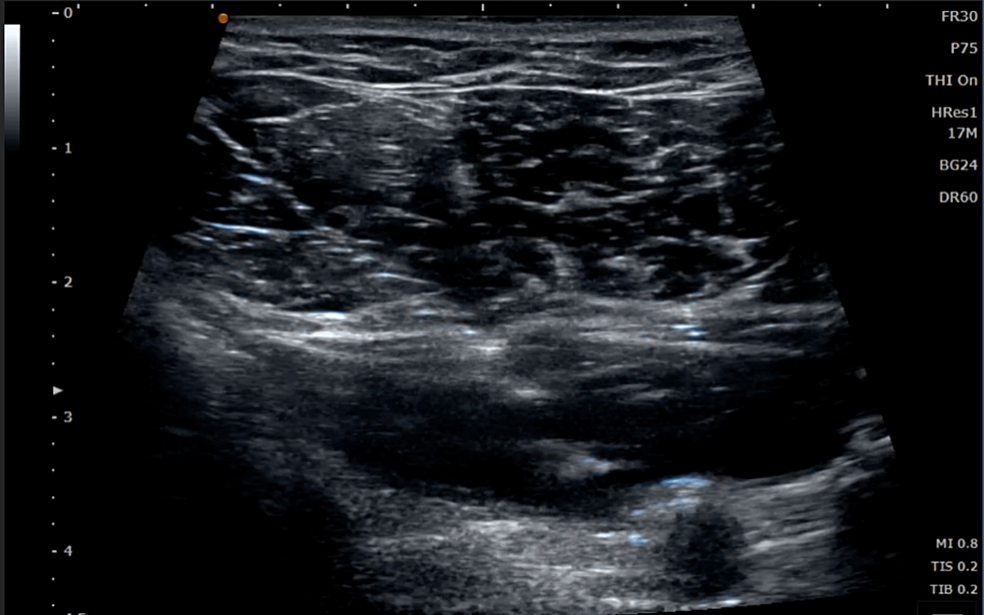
Ultrasound image showing hydrodissection of the patient’s brachial artery in subcoracoid space beneath the pectoralis muscles
